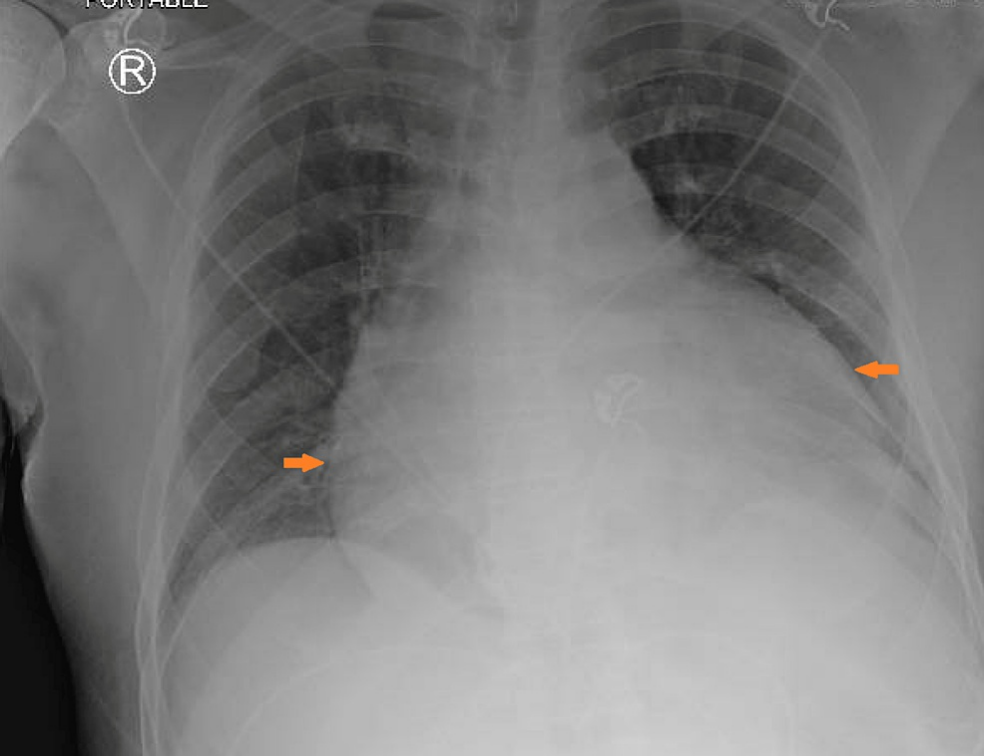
Chest X-ray at the time of initial presentation demonstrating cardiomegaly (arrows)
